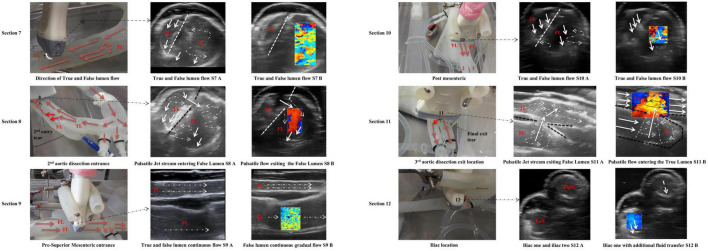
Monitored flow patterns by ultrasound for Sections 7–12.
